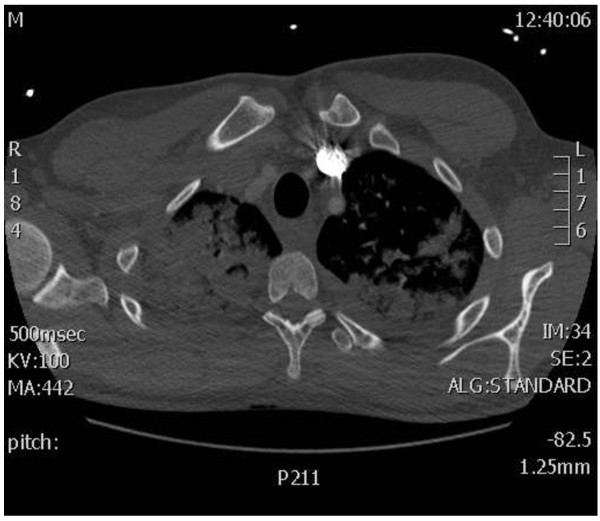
Contrast-enhanced computed tomography scan of the chest. Note the diffuse bilateral infiltrates in the lungs.
